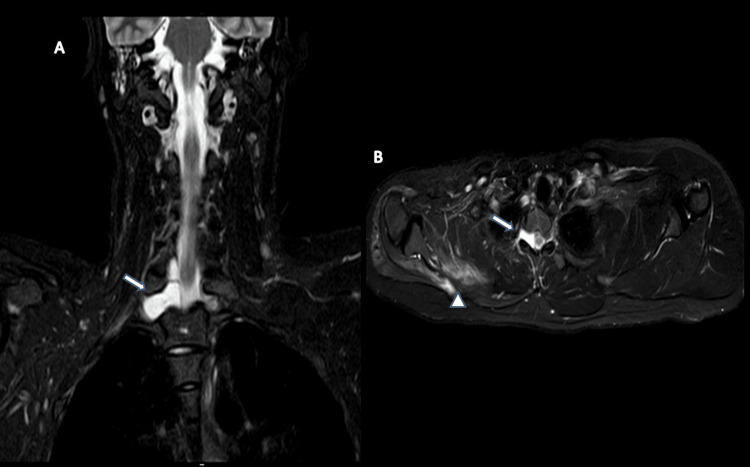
MRI-brachial plexus (A) STIR coronal section, (B) STIR axial section of case 1 MRI: Magnetic resonance imaging; STIR: short tau inversion recovery; CSF: cerebrospinal fluid MRI of the brachial plexus (A) STIR coronal section showing extradural, CSF-filled collection extending to the right neural foramina at the levels of the T1-T2 vertebrae, indicated by a large white arrow. (B) STIR axial section shows a CSF-filled structure extending into the right neural foramen at the levels of the C7-T1 vertebrae, indicated by a large thick white arrow. Shoulder and chest wall muscles, including the supraspinatus, teres, subscapularis, deltoid, pectoralis major and minor, and trapezius, shows signs of mild atrophy compared to the contralateral side accompanied by fatty infiltration and edema-like characteristics, highlighted by a small thick white arrowhead.
